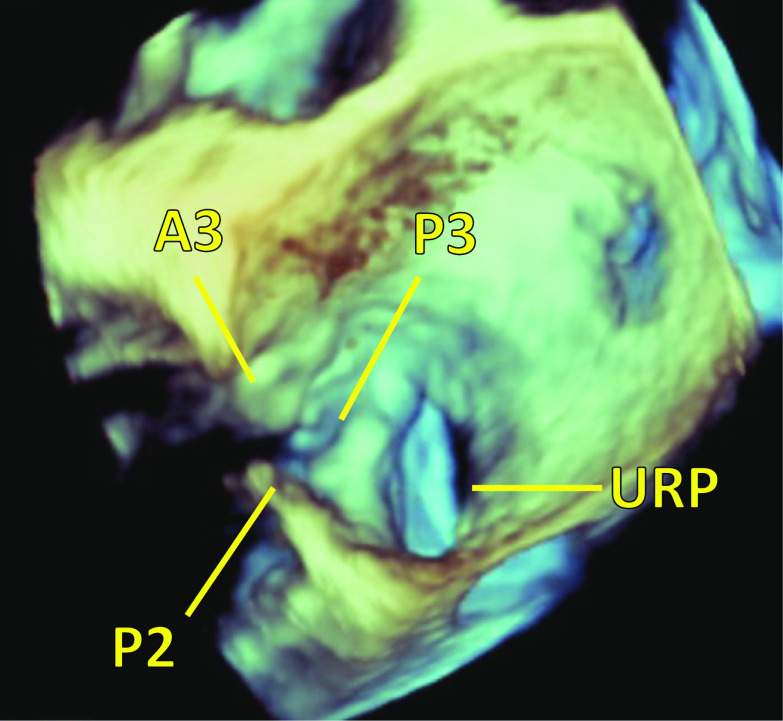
Preoperative three-dimensional transesophageal echocardiography. Preoperative three-dimensional transesophageal echocardiography showed there was an unroofed portion (20 × 10 mm) at the atrial side between segments P2 and P3 of the posterior mitral leaflet. *P2* P2 segment of posterior mitral leaflet, *P3* P3 segment of posterior mitral leaflet, *A3* A3 segment of anterior mitral leaflet, *URP* unroofed portion
